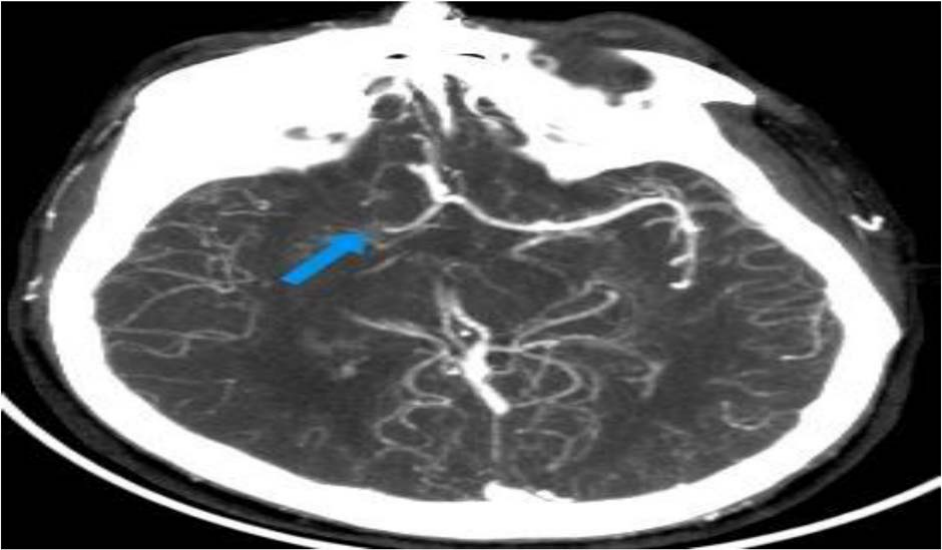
Axial CTA image of the brain showing abrupt cutoff of the right middle cerebral artery (MCA) at the M1 segment (arrow), with reduced opacification of its distal cortical branches. These findings are suggestive of a right MCA occlusion.
